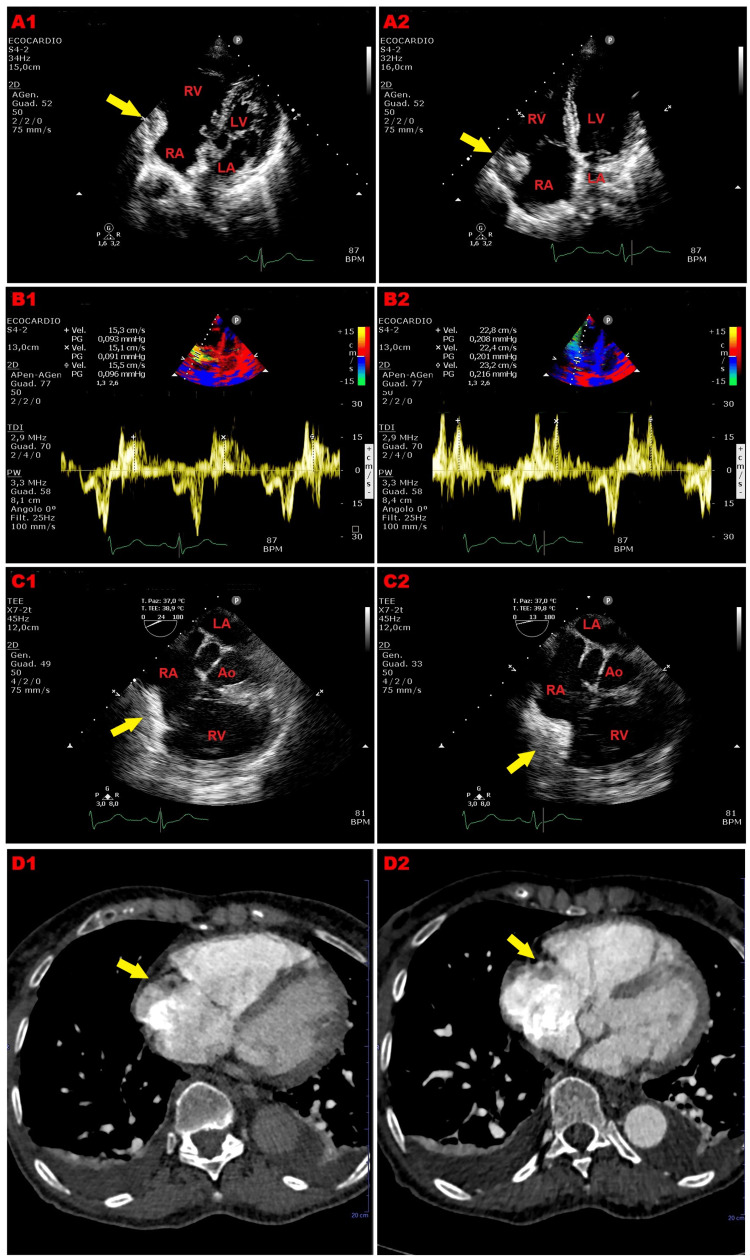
Multi-instrumental evaluation of the suspected right atrial mass. Panel A1: Transthoracic echocardiography. Apical four-chamber view focused on the right-sided chambers (end-diastolic frame), showing moderate dilatation of right heart chambers cavity sizes and significant hypertrophy and hyperechogenicity of the anterior right atrial free wall in proximity of the antero-lateral edge of the tricuspid annulus (yellow arrow). Panel A2: Transthoracic echocardiography. Apical four-chamber view focused on the right-sided chambers (systolic frame), revealing a suspected right atrial mass, occupying the infero-lateral portion of right atrial cavity (Figure 1, Panel A2, yellow arrow). Panel B1: Peak antegrade velocities of the suspected right atrial mass, obtained by placing a 5-mm sample volume of PW-TDI at the level of the mobile portion of the same mass, at end-diastole. Panel B2: Peak antegrade velocities of the suspected right atrial mass, obtained by placing a 5-mm sample volume of PW-TDI at the level of the mobile portion of the same mass, in mid-systole. Panel C1: Mid-esophageal view 24° focused on the right-sided chambers (end-diastolic frame), showing lipomatous hypertrophy involving the anterior right atrial free wall portion near the antero-lateral edge of tricuspid annulus (yellow arrow). Panel C2: Mid-esophageal view 13° focused on the right-sided chambers (mid-systolic frame), revealing infolding of the lipomatous right atrial free wall toward the right atrial cavity (yellow arrow). Panel D1: Contrast-enhanced axial CT scan, confirming both the enlargement of the right heart chambers and the lipomatous hypertophy of the anterior right atrial free wall, which was homogenously hypodense (yellow arrow). Panel D2: Contrast-enhanced axial CT scan, showing the systolic infolding of the lipomatous anterior right atrial free wall toward the cavity (yellow arrow). Ao, aorta; CT, computed tomography; LA, left atrium; LV, left ventricle; PW, pulsed wave; RA, right atrium; RV, right ventricle; TDI, tissue Doppler imaging
